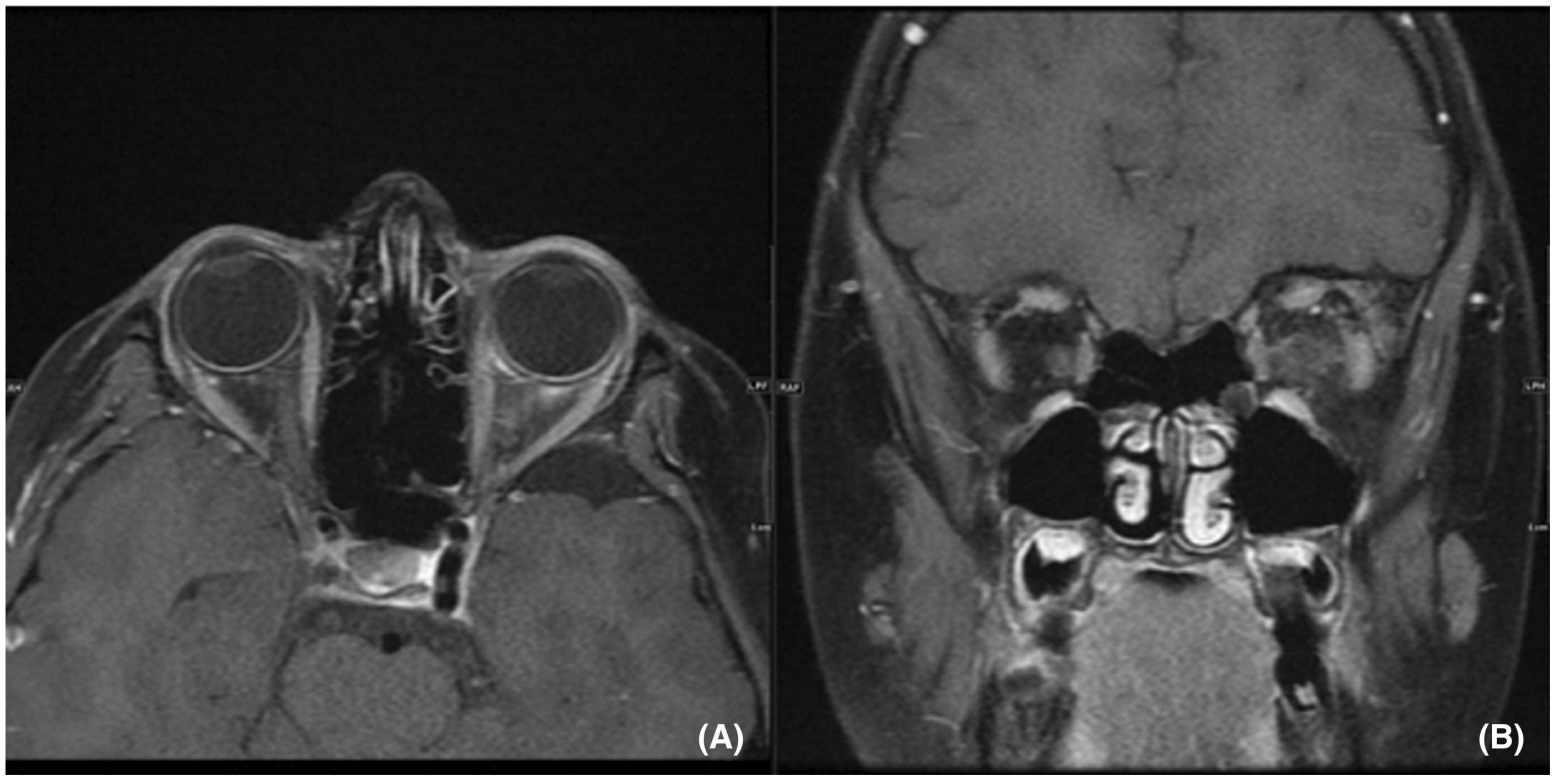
Contrast‐enhanced MR images of the orbit axial (A) and coronal (B) images show diffuse enhancement of the left lateral rectus as well as its tendinous insertion. Mild adjacent stranding of the orbit fat noted in keeping with associated cellulitis.
